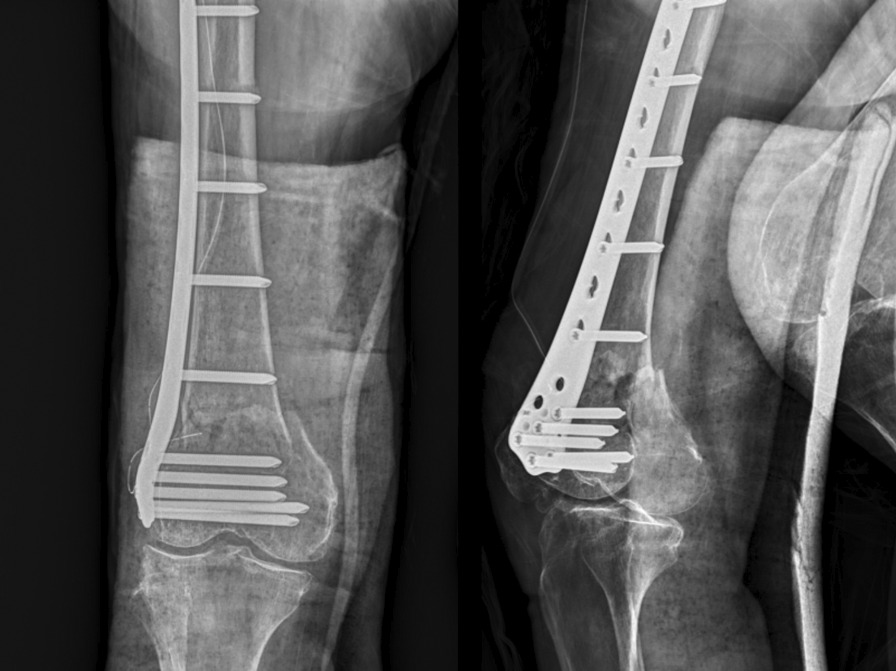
Postoperative radiograph showed well reduced alignment without joint incongruity and varus deformity
